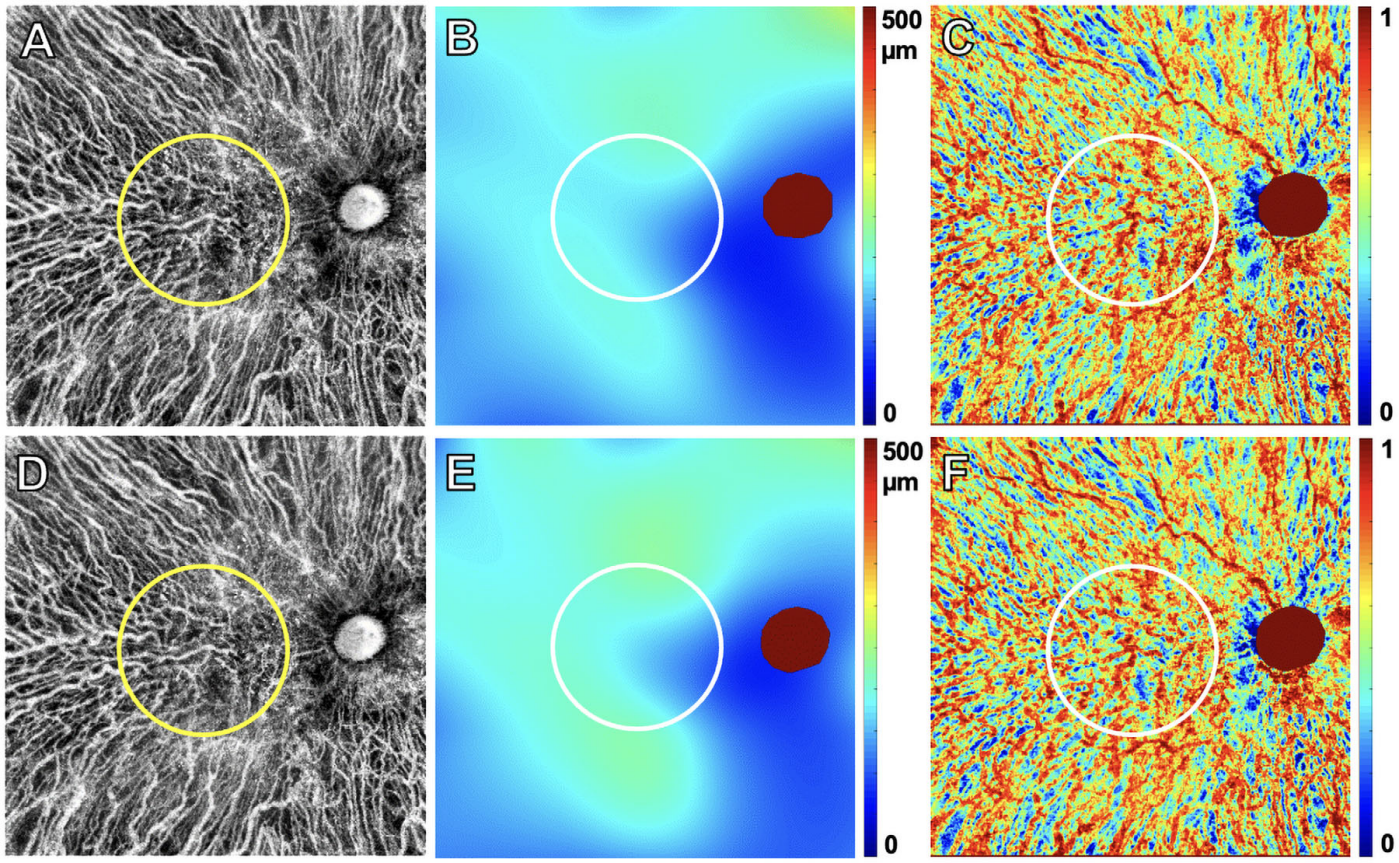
Choroidal vasculature and CT results from SS-OCT 12 × 12-mm scans from the same nonexudative case that did not develop exudation during the follow-up period and the same visits shown in Figure 1. The top row images are from the penultimate visit prior to the last visit before the patient was followed for at least 6 consecutive months without exudation. The bottom row images are from the last visit prior to 6 consecutive months without exudation. The interval between these two visits was 84 days. Choroidal vasculature maps at visit 1 (A) and at visit 2 (D). The *circle* indicates the 5-mm circle centered on the fovea that was analyzed from these scans. CT maps at visit 1 (B) and at visit 2 (E); MCT in the 5-mm circle at visit 1 = 169.66 µm and at visit 2 = 182.18 µm. CVI maps at visit 1 (C) = 0.62 and at visit 2 (F) = 0.62.
